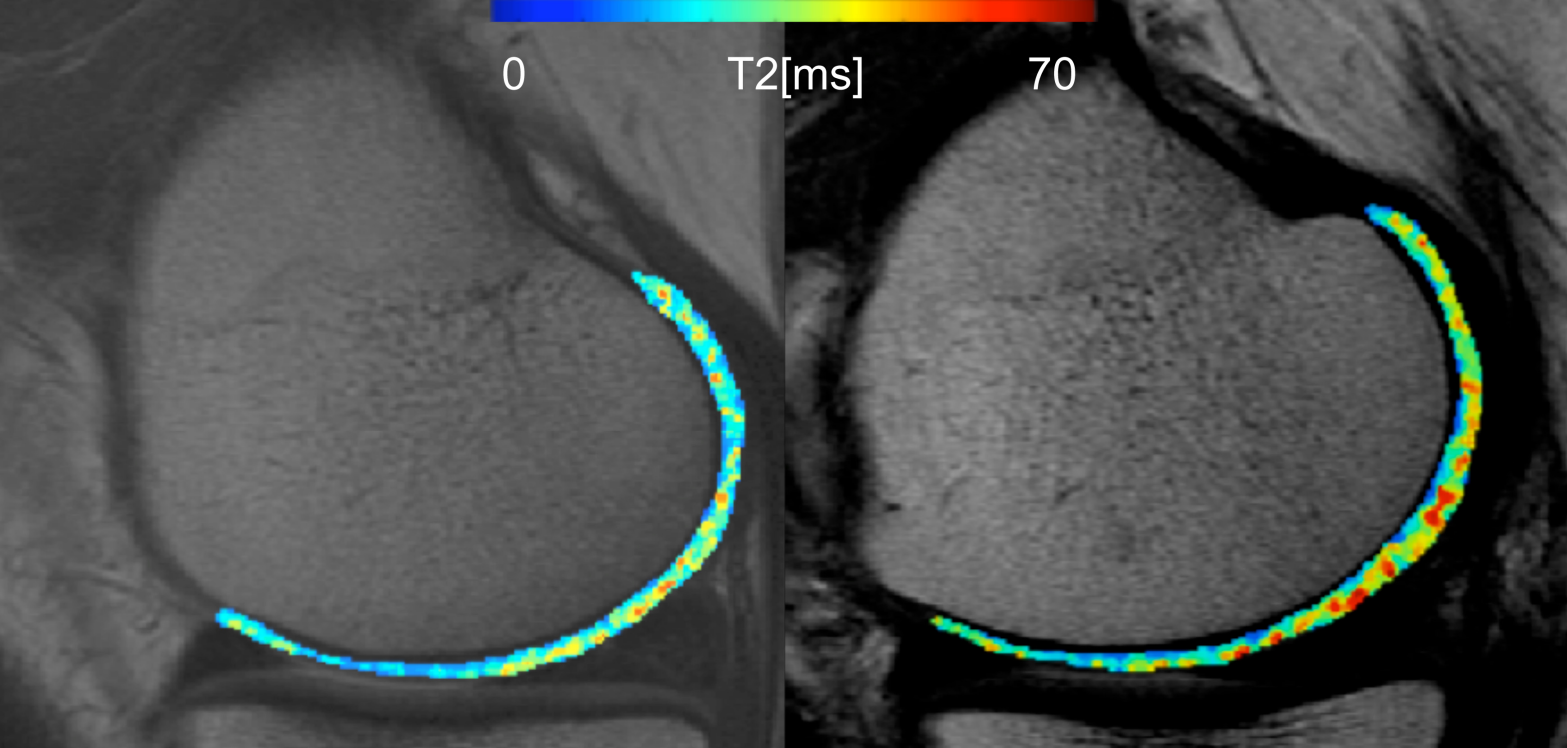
Representative T_2 _maps from a subject from the control cohort (left) and a subject from the incidence cohort (right). Cartilage T_2 _maps are median-filtered with a 3 × 3 kernal for visualization. Both subjects have no cartilage abnormalities (cartilage whole-organ magnetic resonance imaging scores (WORMS) = 0) and no pain (Western Ontario and McMaster Universities Osteoarthritis Index pain = 0); however, the subject from the incidence cohort has elevated mean T_2 _(39.12 versus 33.39 ms), elevated grey level co-occurrence matrix (GLCM) variance (311.63 versus 190.50), elevated GLCM contrast (466.16 versus 266.82), and elevated GLCM entropy (7.17 versus 6.80). Also, the control subject has intrasubstance abnormalities in the medial posterior meniscus (meniscus WORMS = 1). All other meniscus regions had no abnormalities (meniscus WORMS = 0).
